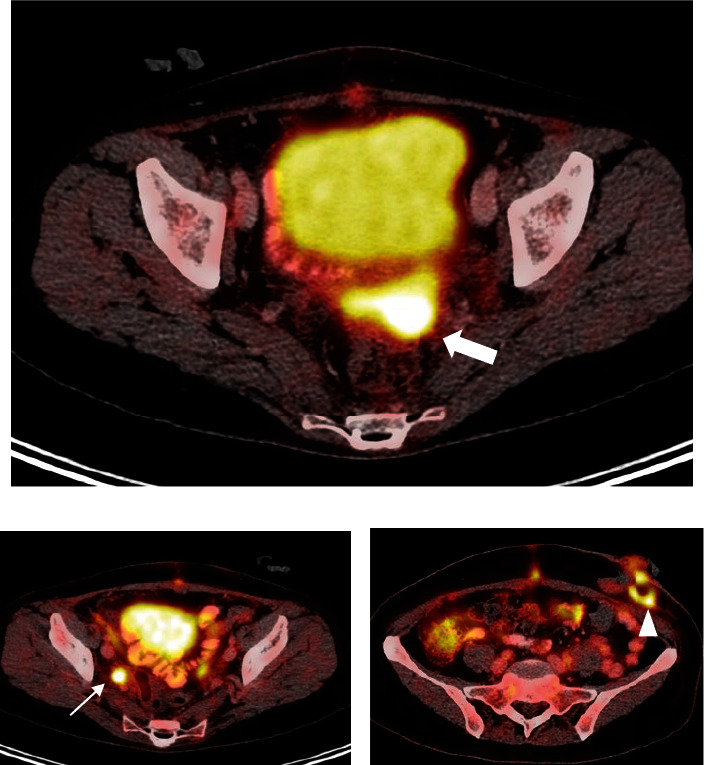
Positron emission tomography/computed tomography (PET-CT) July 2022. The white arrow indicates hot uptake in the vaginal dome (a), the thin arrow shows right adenopathy (b), and the arrowhead shows hypermetabolism at the stoma site compatible with an infectious process (c).
